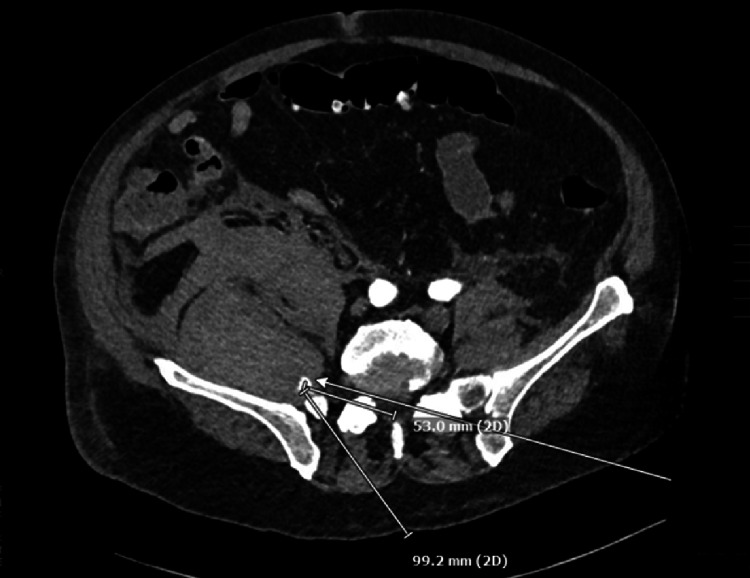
Computed tomography (CT) scan. The "contrast blush" sign (arrow) demonstrates the location of the source of the bleed.
